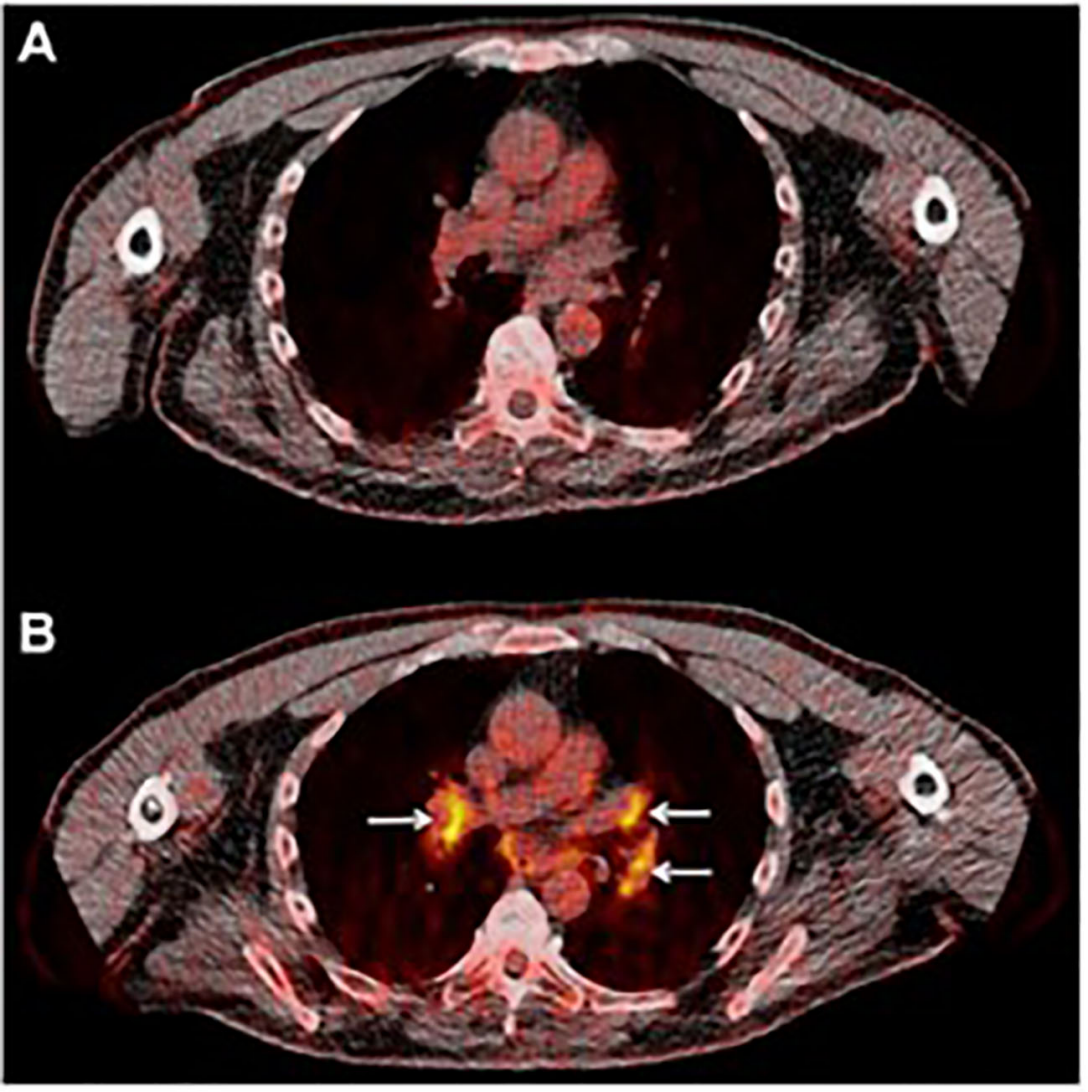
Sarcoid-like lymphadenopathy. (A) Transaxial FDG image of the thorax of a metastatic melanoma patient prior to starting immunotherapy (B) After two cycles of anti-CTLA-4 therapy FDG avidity in the mediastinal and hilar lymph nodes was evident. Adapted with permission from Springer Nature:Sachpekidis C, Larribere L, Kopp-Schneider A, Hassel JC, Dimitrakopoulou-Strauss A. Can benign lymphoid tissue changes in (18)F-FDG PET/CT predict response to immunotherapy in metastatic melanoma? Cancer Immunol Immunother. 2019;68:297-303.
